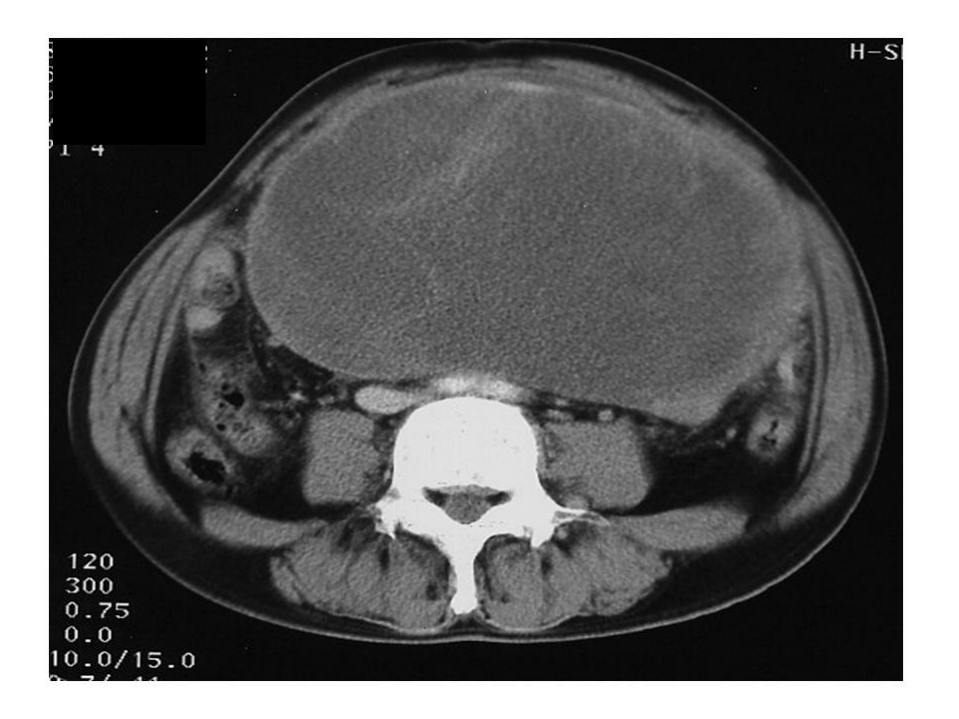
Contrast-enhanced CT revealed a large tumor in the entire abdomen and showed septum in the cystic tumor.
